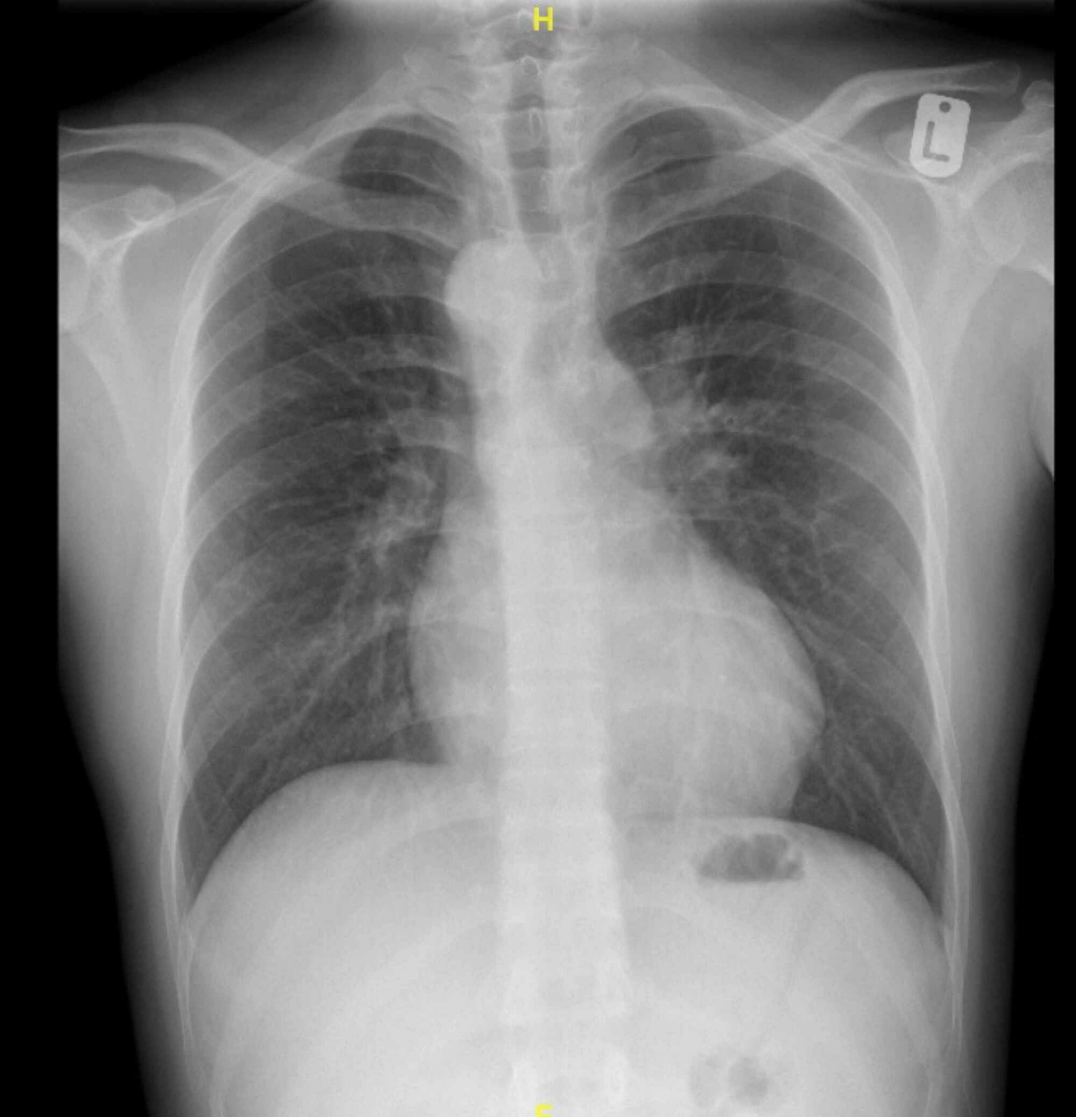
Patient’s chest X-ray demonstrating normal appearance of the heart.
